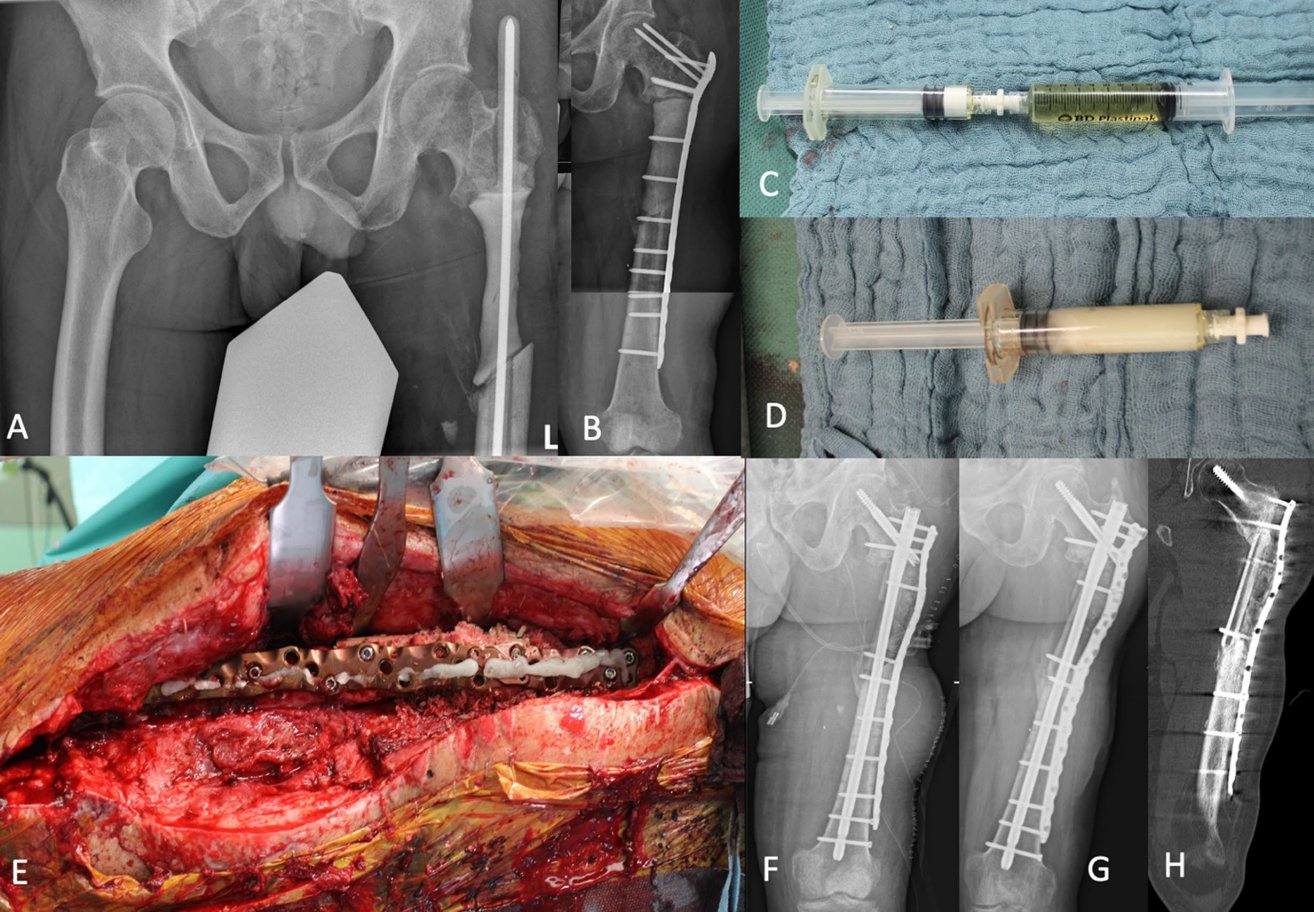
Subtrochanteric segmental bone defect of the left femur was temporarily stabilized with an intramedullary custom-made PMMA bone cement-coated rod (A). After removal of the rod, a PMMA spacer with colistin was added for dead space management and the bone stabilized with an angular stable plate (B). The second stage of the Masquelet procedure was performed with an intramedullary nailing and augmentation plate. During bone defect reconstruction phages were applied to the DAC® gel (C,D). The DAC gel loaded with Intesti bacteriophage was finally placed on the silver-coated plate (E). Postoperative X-ray demonstrates the bone defect filled with autograft fibula graft and allogenic cancellous bone (F). After 12 months, sufficient bone healing was confirmed by performing X-ray (G) and computed tomography (H).
